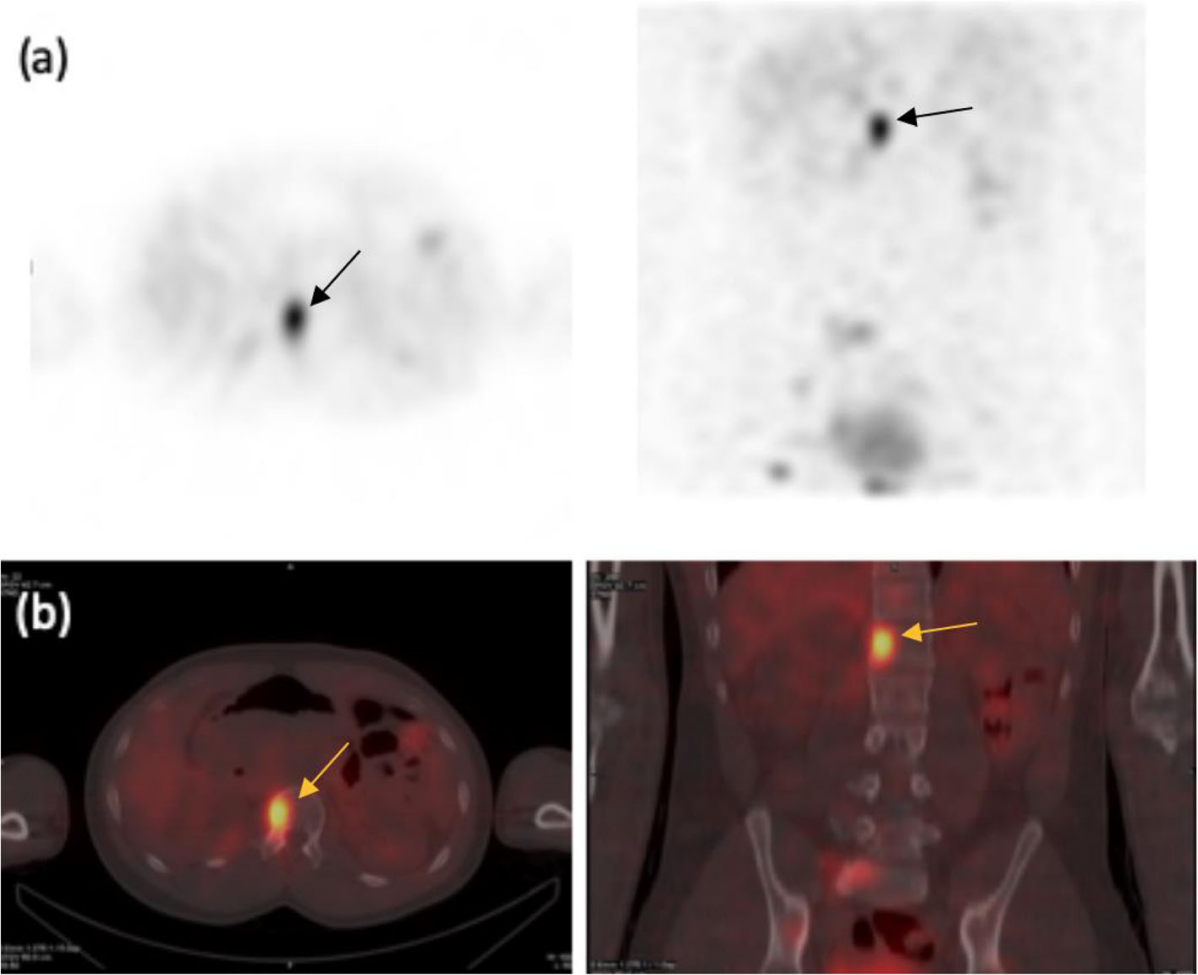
Iodine-123 MIBG image of a NET metastasis shown with (a) SPECT and (b) SPECT/CT. SPECT only images of the metastasis do not provide anatomical information, while the SPECT/CT image provides both localization and complementary CT information
